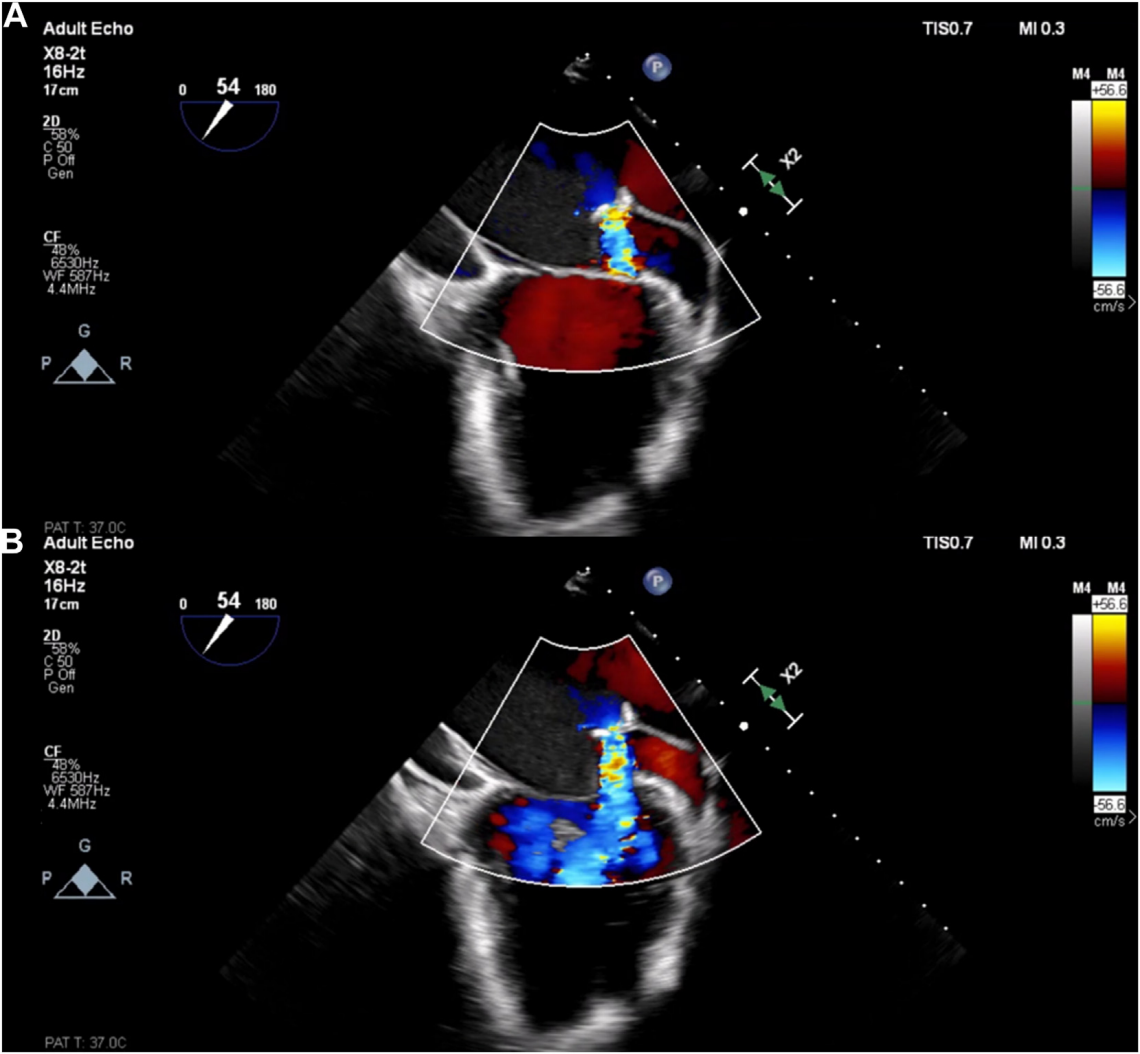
Two-dimensional TEE, midesophageal commissural view (54°), with color flow Doppler in systole (A) and diastole (B), demonstrates the continuous flow across the cor triatriatum membrane aperture within the left atrium.
